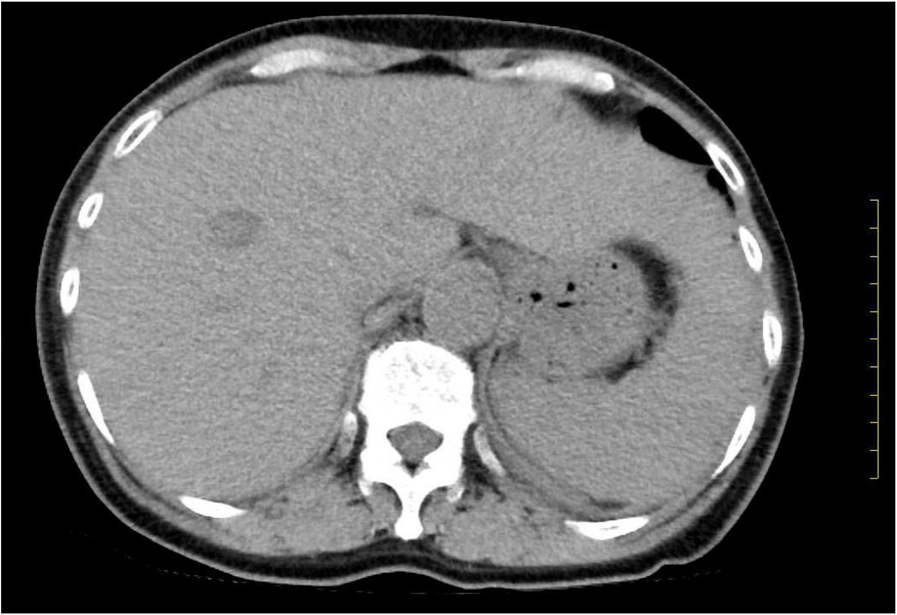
Abdominal computed tomography (CT) showing splenohepatomegaly (major axis of the spleen, 10.5 cm; size of the right hepatic lobe, 15.0 cm; size of the left hepatic left lobe, 12.0 cm)
